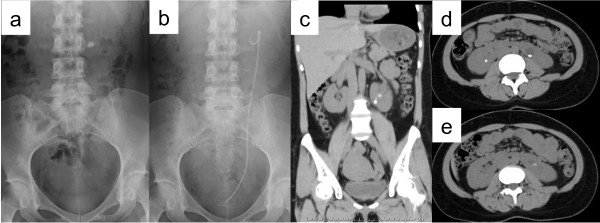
Case 2: (a) preoperative and (b) postoperative kidneys, ureters, and bladder X-ray films. Preoperative non-contrast (c) coronal and (d, e) axial computed tomography images.
